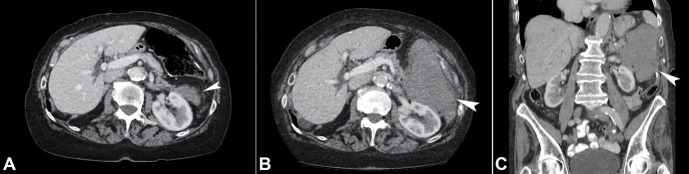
Abdominal CT – A – (axial view) showing a 4.7 x 3.0cm soft-tissue density mass anterior to the left kidney, adjacent to the tail of the pancreas and the previous colonic anastomosis at the splenic flexure; B – (axial view) showing the rapid expanding tumor, 12.7 x 8.6cm, adherent to the nearby structures; C – (coronal view) showing the rapid expanding tumor, 12.7 x 8.6cm, adherent to the nearby structures.
